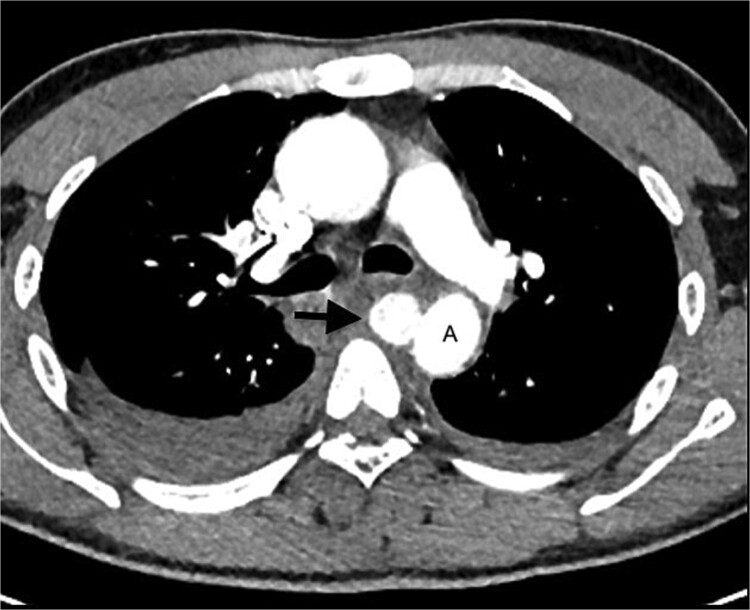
CT angiogram of the chest confirms a pseudoaneurysm (arrow) of the thoracic aorta (A), with surrounding mediastinal haematoma and bilateral haemothoraces.
